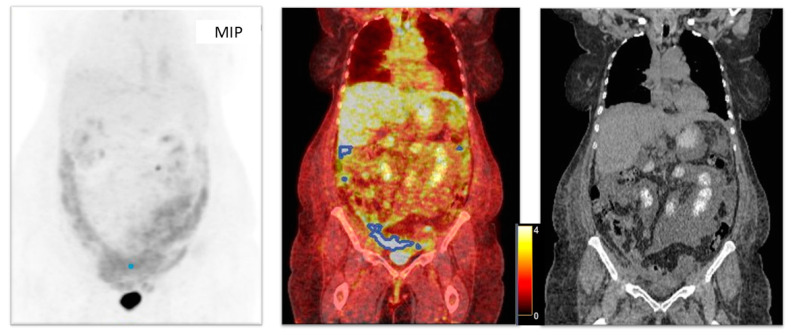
Example of exclusion criteria: non-target volume 0. Only one VOI was semiautomatically drawn (blue dot and lines) and categorized as target. Thus, the non-target volume was zero. The patient was excluded, since it was not possible to apply our methodology. MIP: maximum intensity projection. Blue line indicates the volume of interest (VOI). Blue dot: locations of the VOIs. Color bar indicates SUVmax values.
